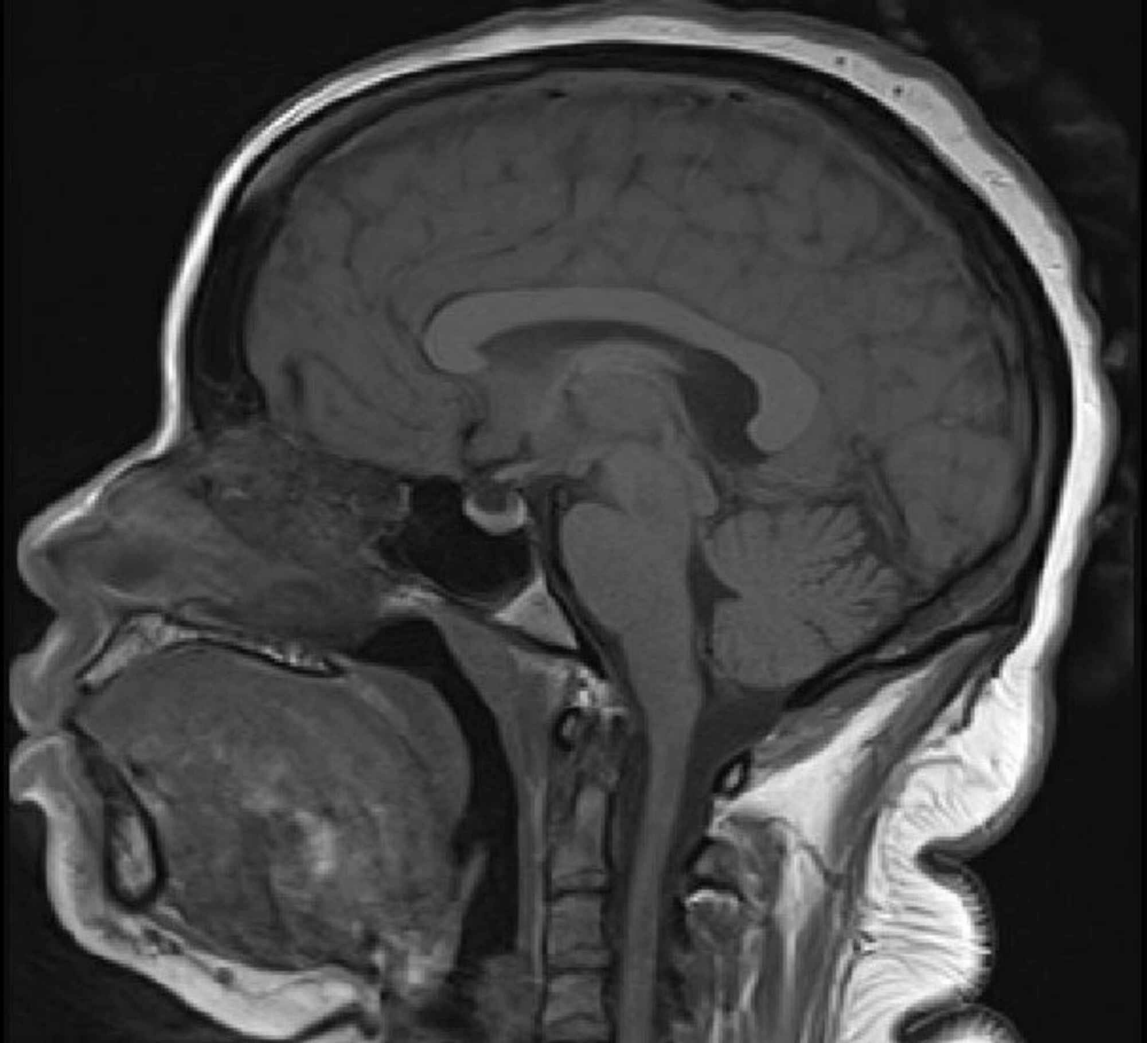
T1-weighted sagittal MRI of the brain
